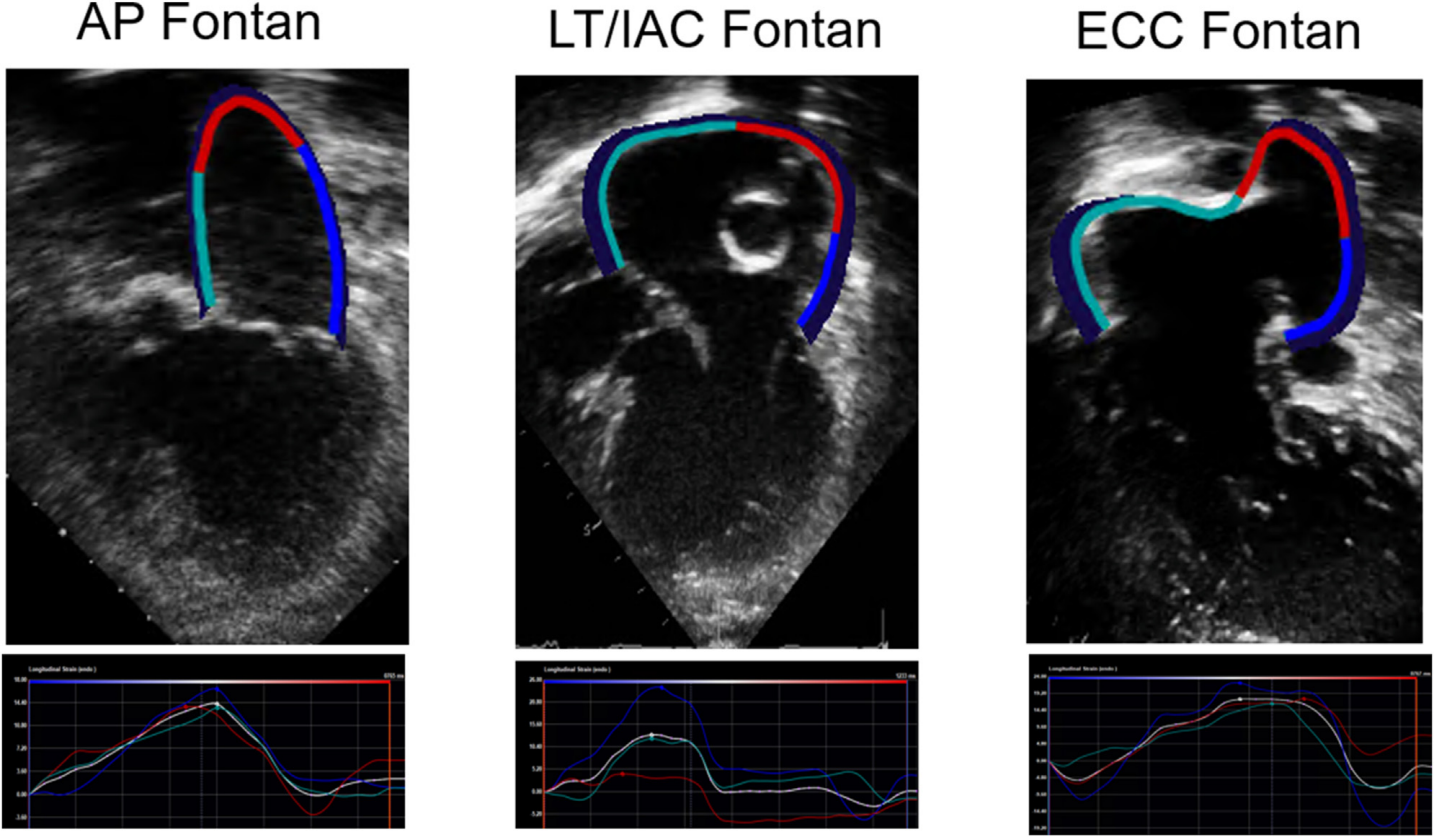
Top: representative echocardiographic images of pulmonary venous atrial strain analyses from a patient with atriopulmonary Fontan (AP), lateral tunnel/intra-atrial conduit (LT/IAC), and extracardiac conduit (ECC) Fontan. Reservoir strain was measured at the end of ventricular systole, booster strain was measured at the end of atrial systole, and conduit strain was calculated as the difference between reservoir strain and booster strain. All strain measurements were made using the QRS as the fiduciary point.
